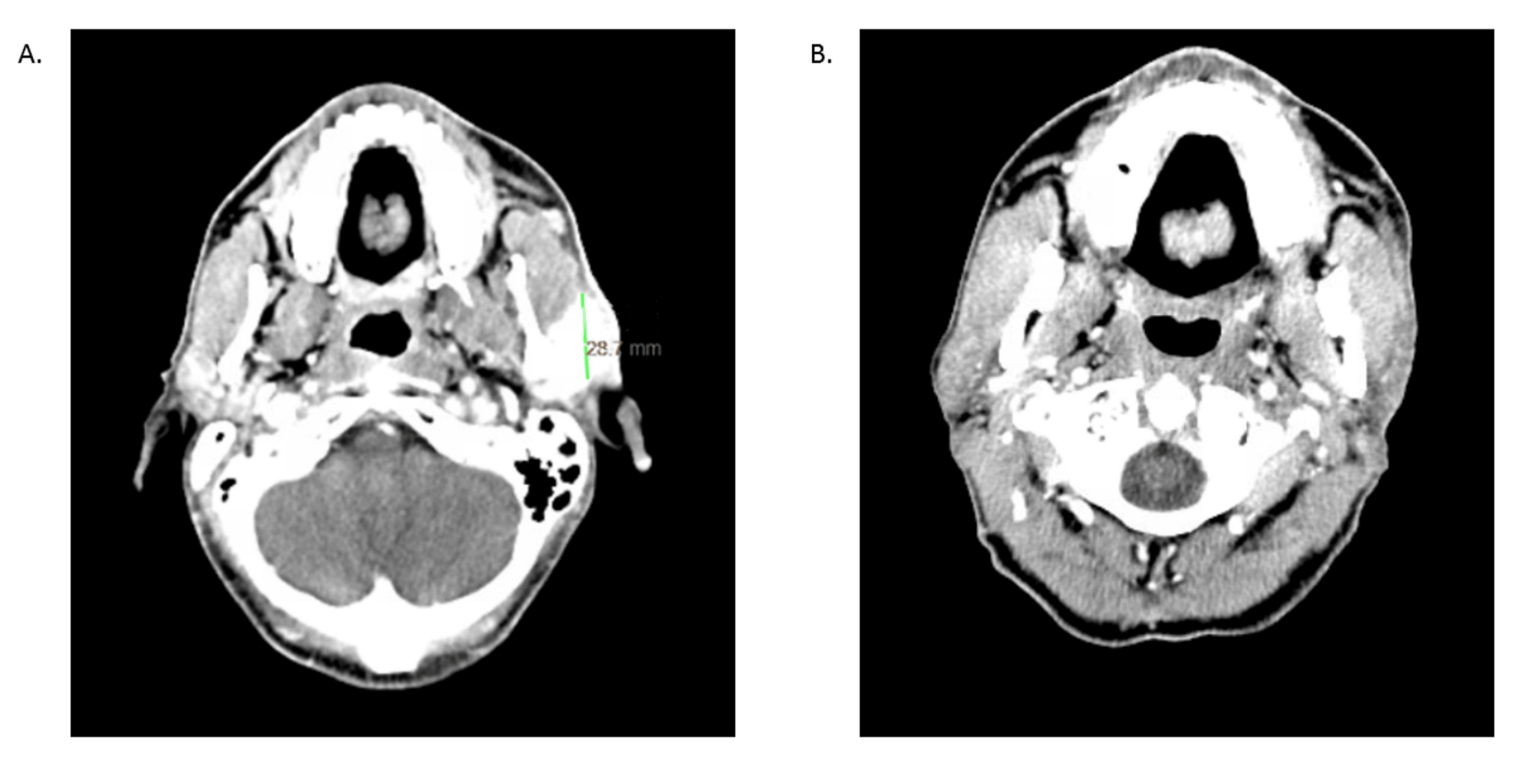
Computed tomography scans A. Baseline computed tomography (CT) scan with biopsy-proven recurrence showing a 2.9 cm mass. B. First CT scan for disease evaluation after pembrolizumab initiation showing a complete response to therapy.
